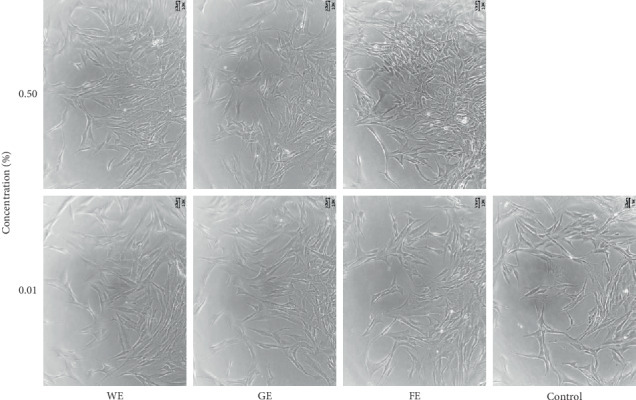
Comparison of the morphologies of human fibroblast cells on the third day after treatment with 0.01% and 0.5% of the three different extracts and comparison with the control (not treated). GE, gel juice extracted after peeling off the leaves of *A. vera*; WE, the water extract from the whole aerial parts of *A. arborescens* at 100°C for 24 hours; FE, fermentation of the whole aerial parts of *A. arborescens* grown with *L. plantarum* at 37°C for 4 days and further extracted by an ultrasonicator at 40 kHz for 1 hour at room temperature.
